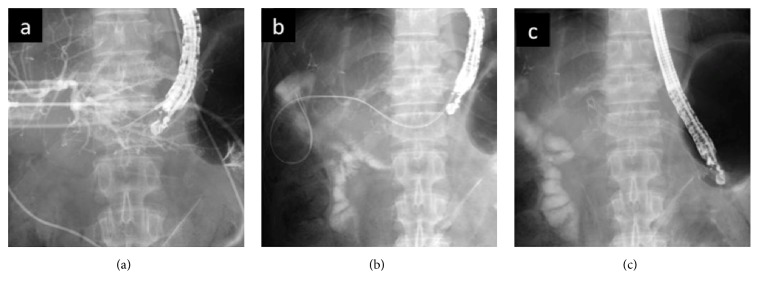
Endoscopic ultrasound-guided hepaticogastrostomy (EUS-HGS). (a) Bile duct is punctured with a 19-G needle. (b) Guidewire is inserted into the bile duct. (c) Metallic stent is placed.
